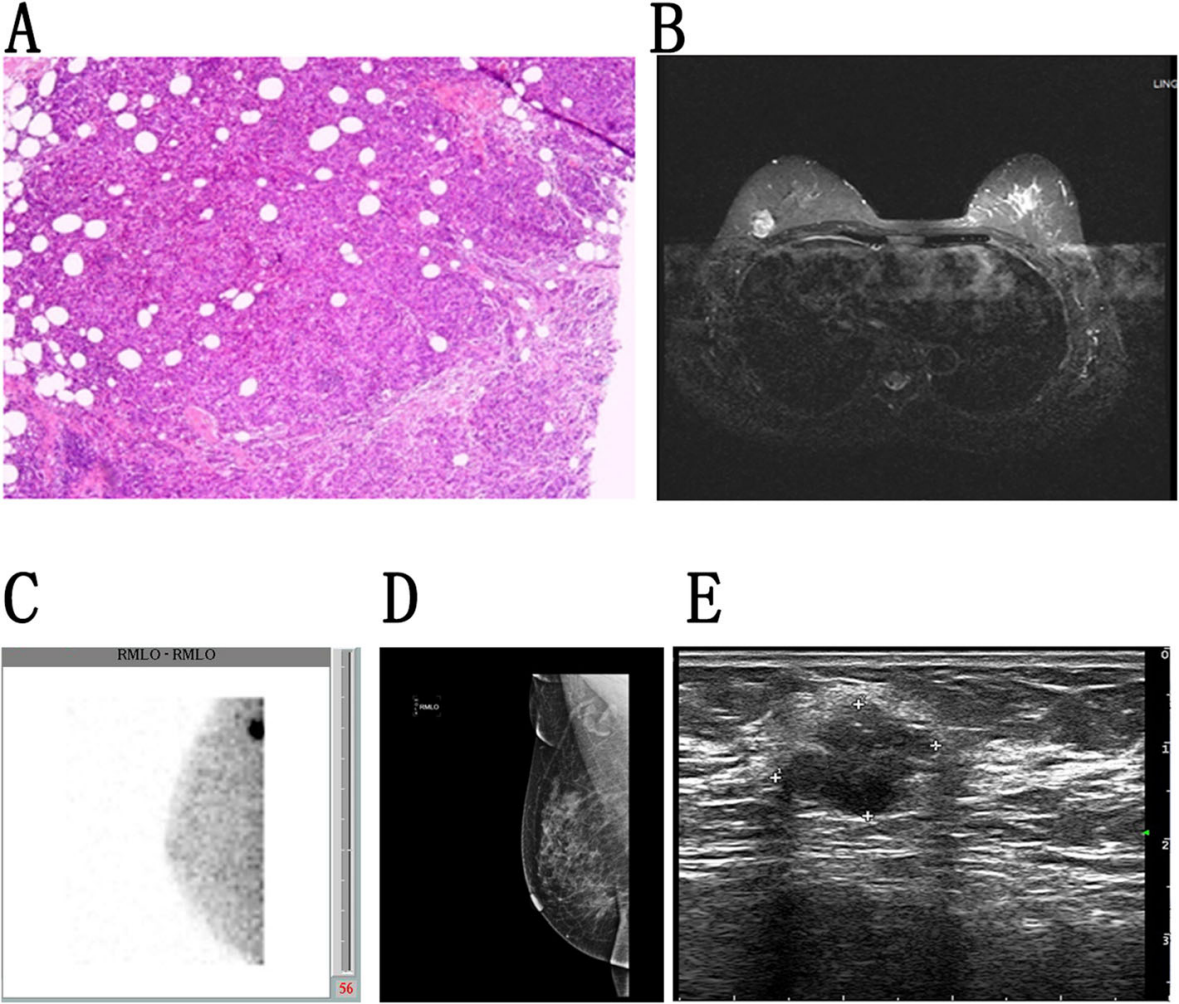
Breast scans from a woman. a. Pathological findings demonstrating invasive ductal carcinomas. b. MRI analysis revealed a nodular mass (BI-RADS 3) in the right breast that was found to be benign (1.2 × 1.4 cm). c. BSGI (RMLO) revealed focal enhanced radiotracer uptake in the right breast that was found to be malignant, LNR = 3.03. d. Mammography (RMLO) revealed breasts of density class C and the presence of a nodular-like (BI-RADS 3) structure in the upper outer quadrant area. e. Ultrasound detected a lobulated hypoechoic lesion (BI-RADS 4A) in the right breast, 1.5 × 1.2 cm
